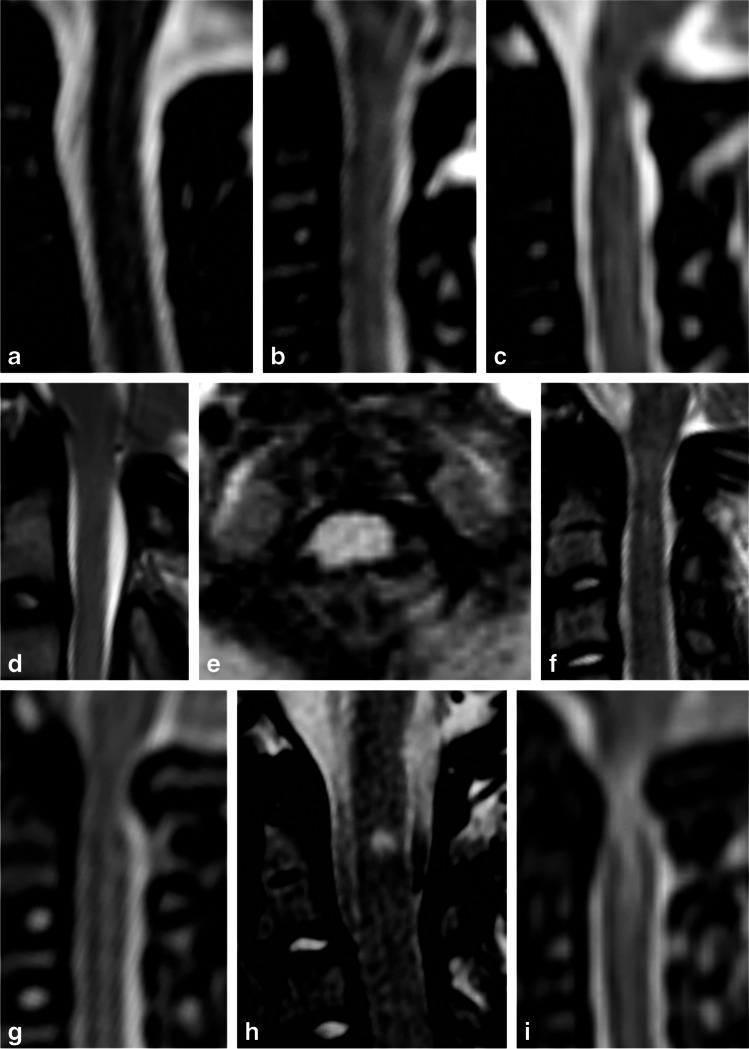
Representative T2 sagittal imaging showing eAFMS; see Table 1 for a description of the findings in each grade. Sagittal (a-d, f-i) and axial (e) T2 magnetic resonance images: a A 23-month-old girl with a normal CCJ. b A 30-month-old girl with AFMS 1 stenosis. c An 8-month-old girl with AFMS 2a stenosis. d A 4-year-old boy with AFMS 2b stenosis. e An 8-month-old girl with AFMS 2c stenosis. f A 7-year-old girl with AFMS 3a stenosis. g A 12-month-old boy with AFMS 3b stenosis. h A 7-year-old girl with AFMS 4a stenosis. i A 9-month-old boy with AFMS 4b stenosis. *AFMS* achondroplasia foramen magnum score, *CCJ* craniocervical junction*, eAFMS* extended achondroplasia foramen magnum score
